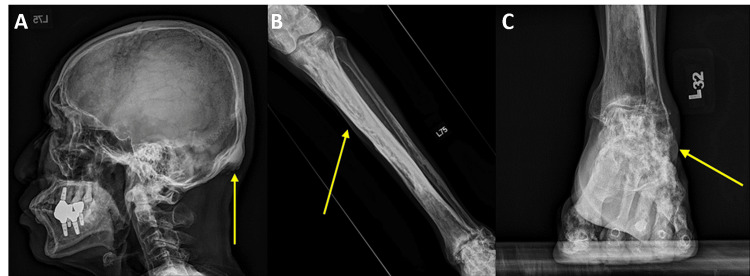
Radiographic findings consistent with melorheostosis. The images show (A) lateral skull view, (B) anteroposterior view of the left tibia, and (C) anteroposterior view of the left ankle. Arrows indicate melorheostosis lesion.
